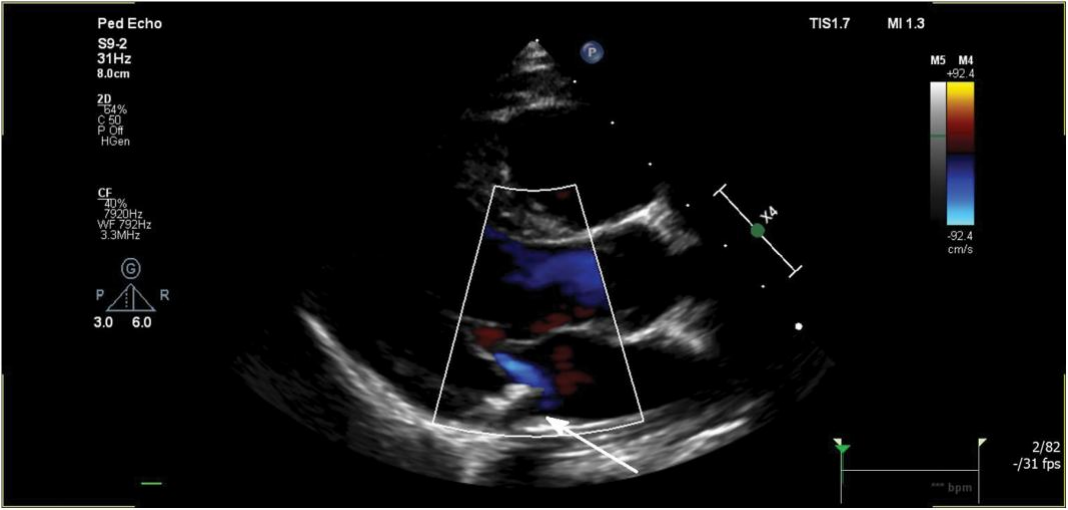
Transthoracic echocardiography, parasternal long-axis view, 15 months after mitral valve reconstruction. Minimal mitral regurgitation, the Gore-Tex ring can be appreciated as an echogenic structure (arrow).
